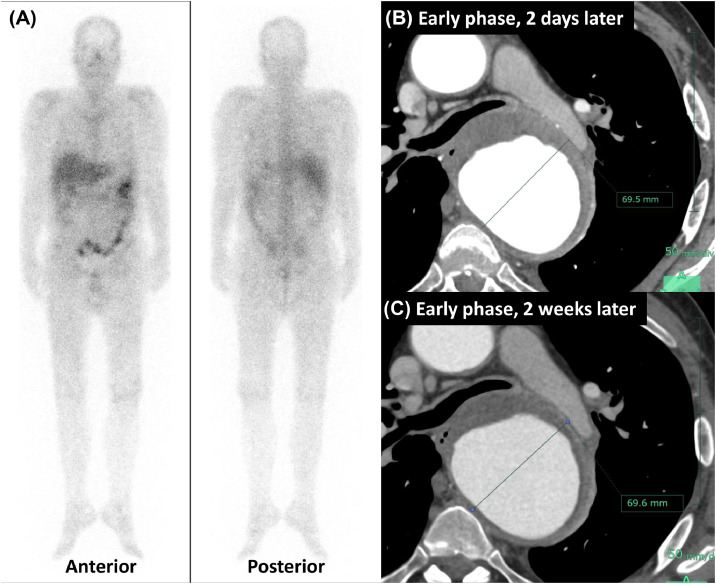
(A) Anterior and posterior whole-body gallium scintigraphy images. No abnormal uptake is observed in the descending thoracic aorta or elsewhere suggestive of active systemic inflammatory disease. (B, C) Follow-up contrast-enhanced CT early-phase images obtained 2 days (B) and 2 weeks (C) after the initial examination. The maximum aneurysm diameter remained unchanged (69.5 mm in [B] and 69.6 mm in [C]), indicating no significant interval enlargement.
